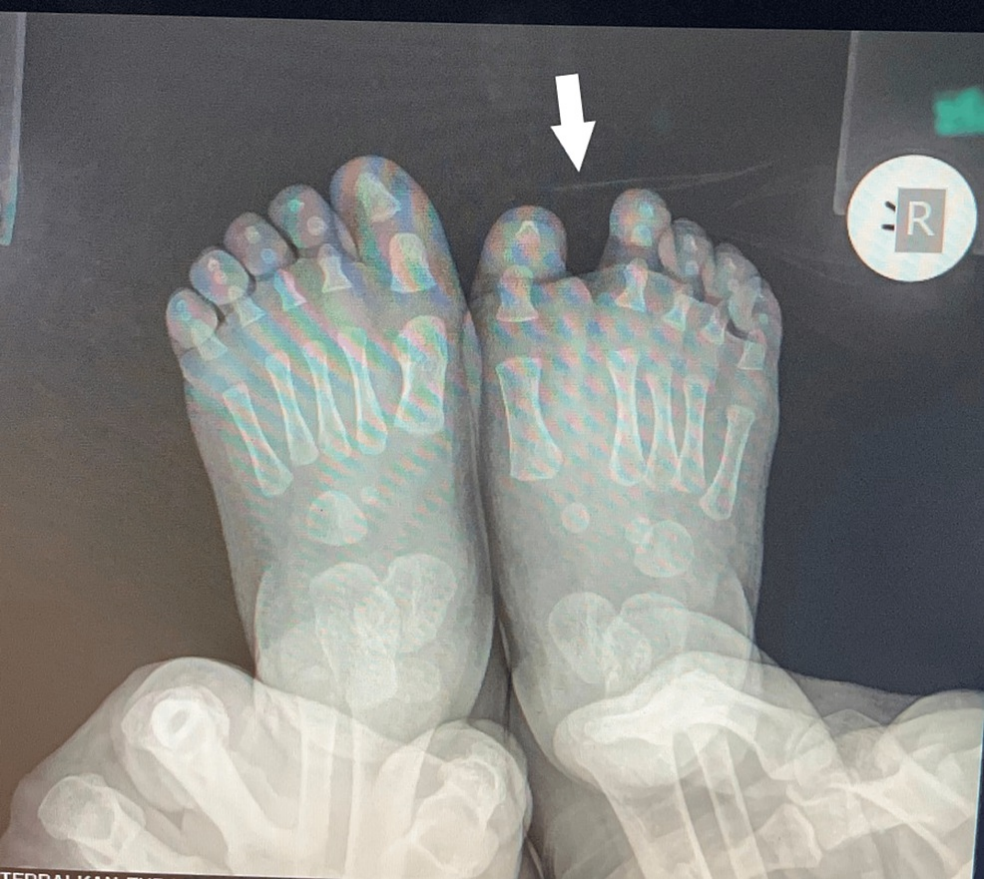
X-ray of the fifth patient's foot six months after the procedure.
